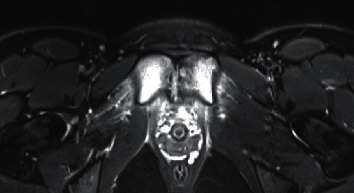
MR image when the patient relapsed with pain.
